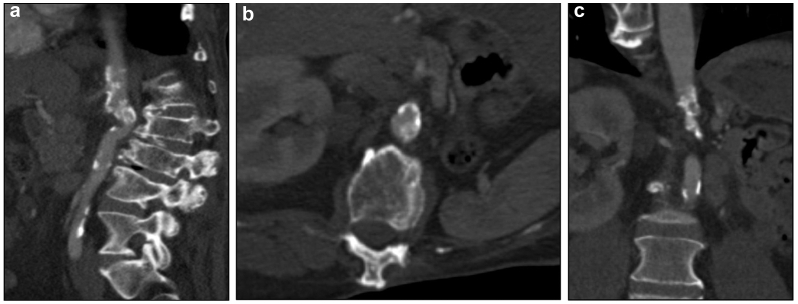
Preoperative computed tomography angiography (CTA) demonstrating high-grade aortic stenosis in the (a) sagittal, (b) axial, and (c) coronal planes.
